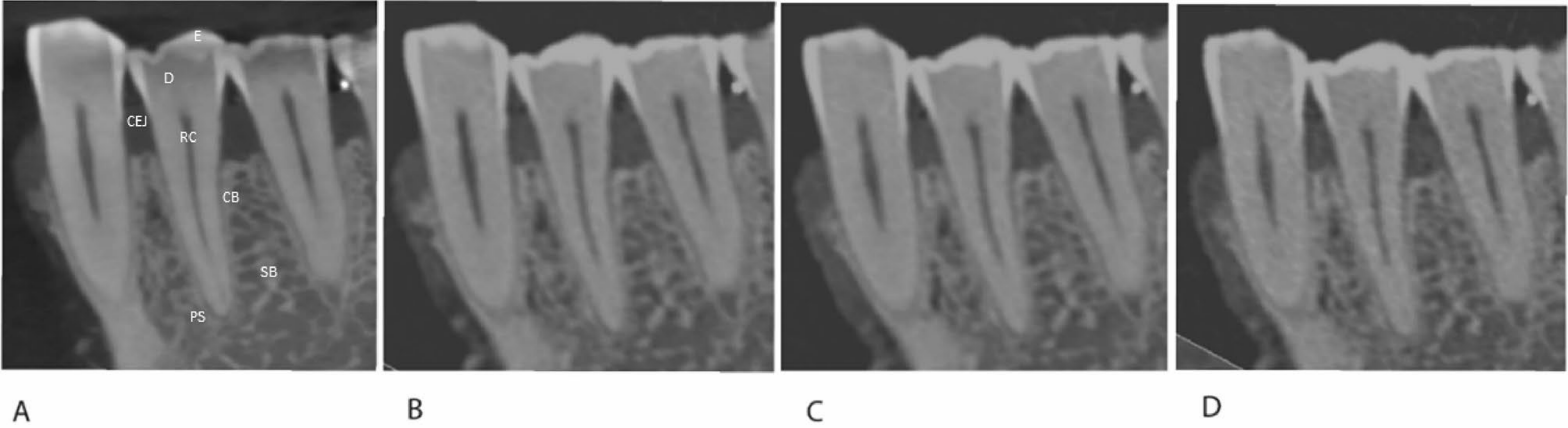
Sagittal reformations of the same region: Teeth 33, 34 and 35 in (A) CBCT (B) PCCT HD (C) PCCT MD (D) PCCT LD; *D* dentine, *E* Enamel, *CEJ* Cemento-Enamel-Junction, *RC* root canal, *PS* periodontal space, *CB* cortical bone, *SB* spongious bone. (C = 1535 HU, W = 8504 HU).
